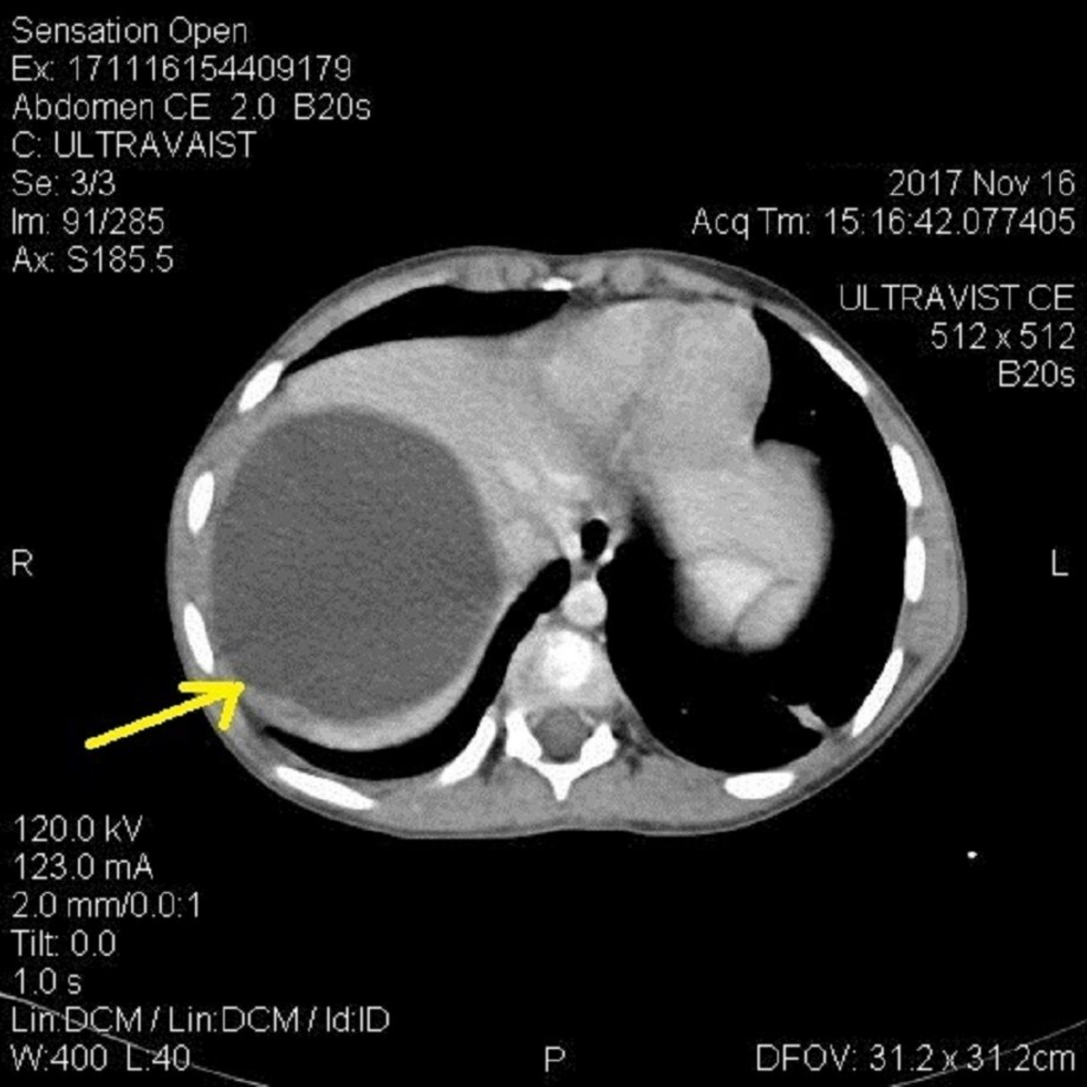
Computed tomography (CT) findings CT abdomen showing large cyst in right lobe subdiaphragmatic area of liver.
